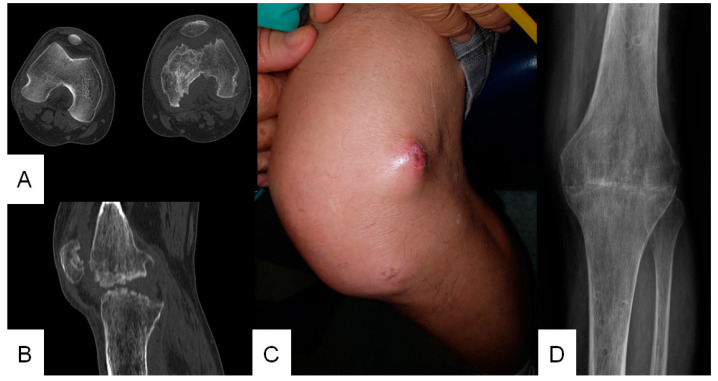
Knee osteoarticular sporotrichosis. (A,B) Computed tomography scan showing destructive osteoarthritis on the left knee (September 2018). (C) Left knee with a nodular sporotrichosis lesion (July 2018). (D) Radiograph of the left knee, post-arthrodesis, revealing degenerative joint disease and femorotibial ankylosis (August 2020).
